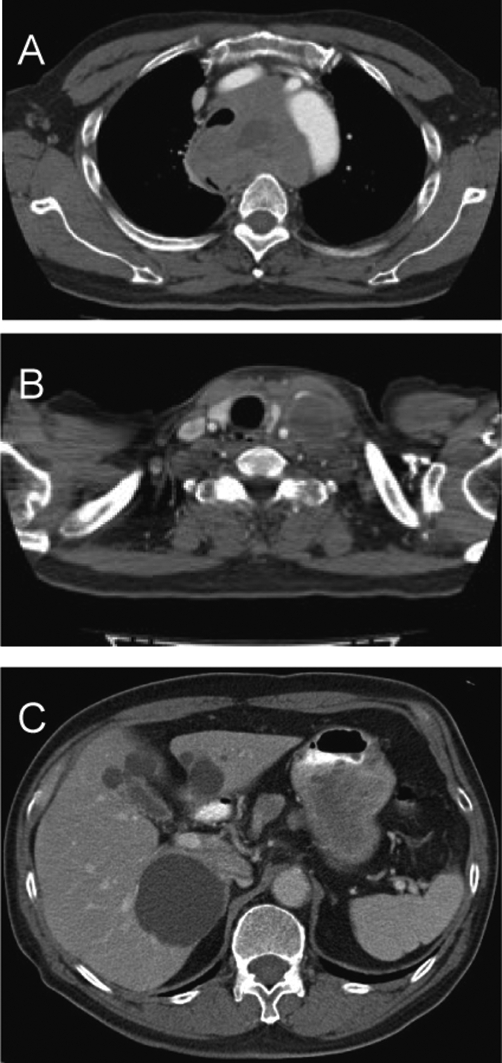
Contrast enhanced CT showed 7.7 cms mediastinal lymph nodes compressing the esophagus and trachea at the level of the aortic arch (A), 3.3 × 3.0 cms left supraclavicular (B) and 1.9 × 1.5 cms abdominal lymph nodes (C).
